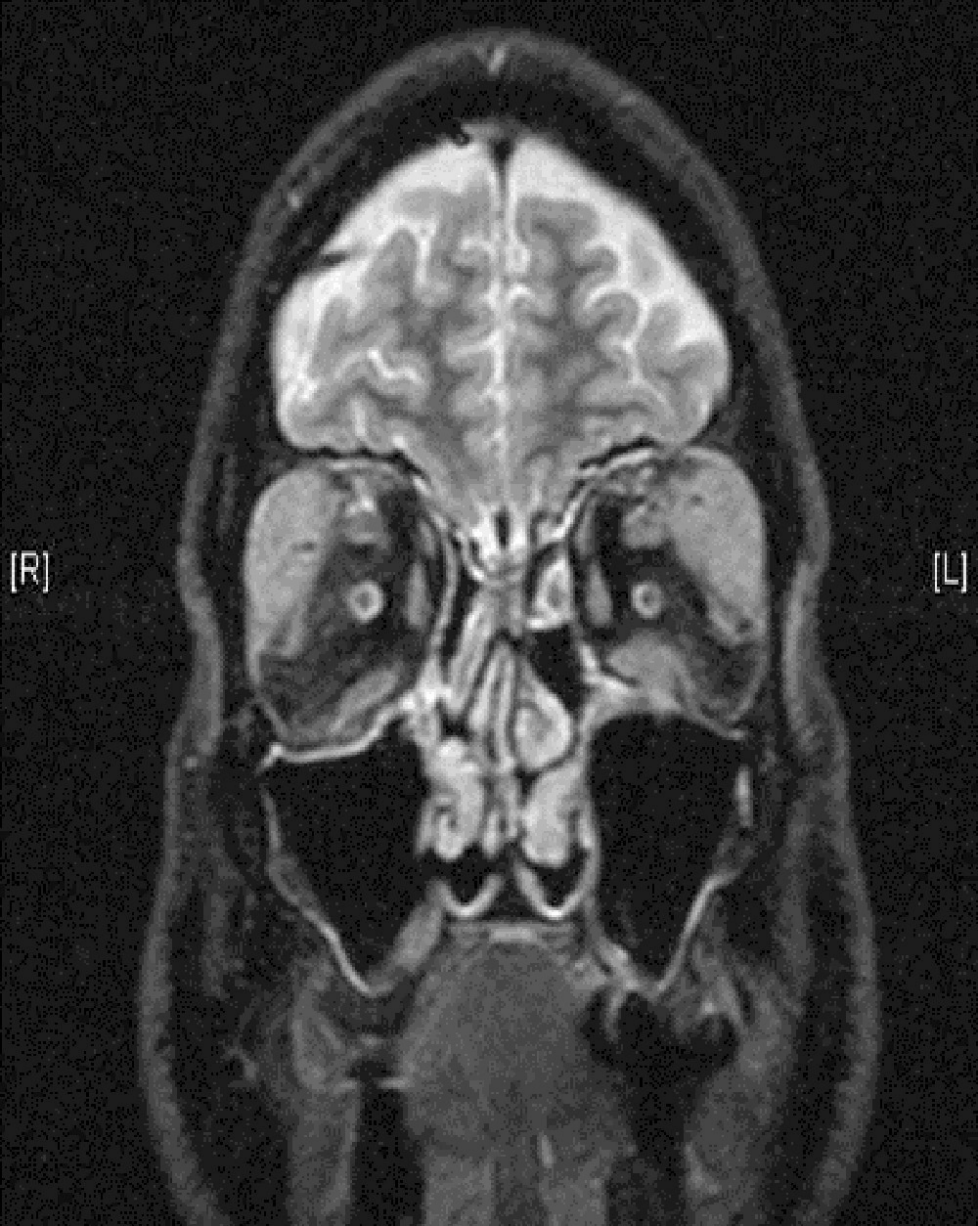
MRI Orbits Gross enlargement of the bilateral lacrimal glands with displacement of the extraocular muscles and globes is seen.
